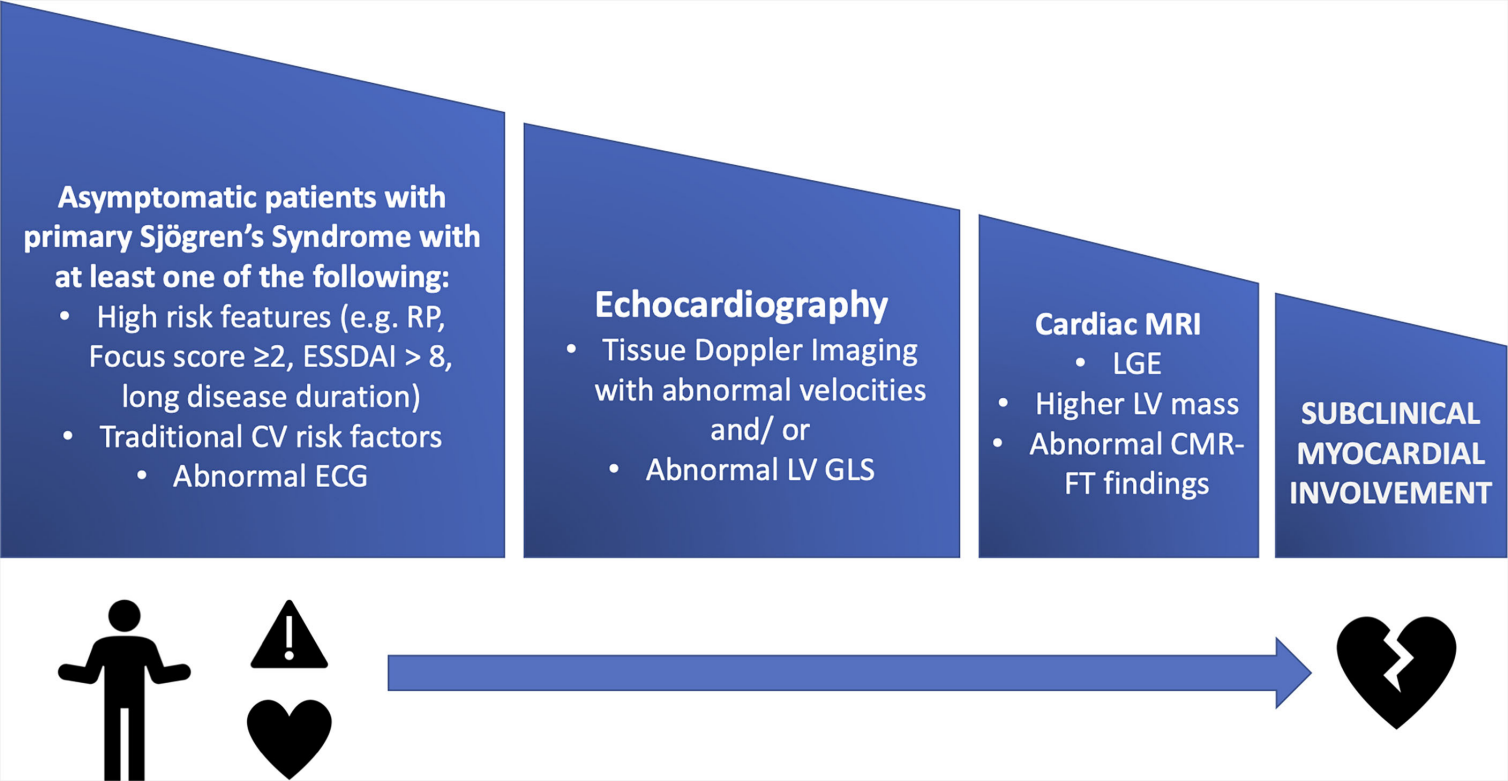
A stepwise approach for screening pSS patients for subclinical myocardial involvement using multimodality imaging. CMR- FT, cardiac magnetic resonance feature tracking; CV, cardiovascular; ESSDAI, EULAR Sjogren’s Syndrome Disease Activity Index; GLS, global longitudinal strain; LGE, late gadolinium enhancement; LV, left ventricle; MRI, magnetic resonance imaging; RP, Raynaud’s Phenomenon.
